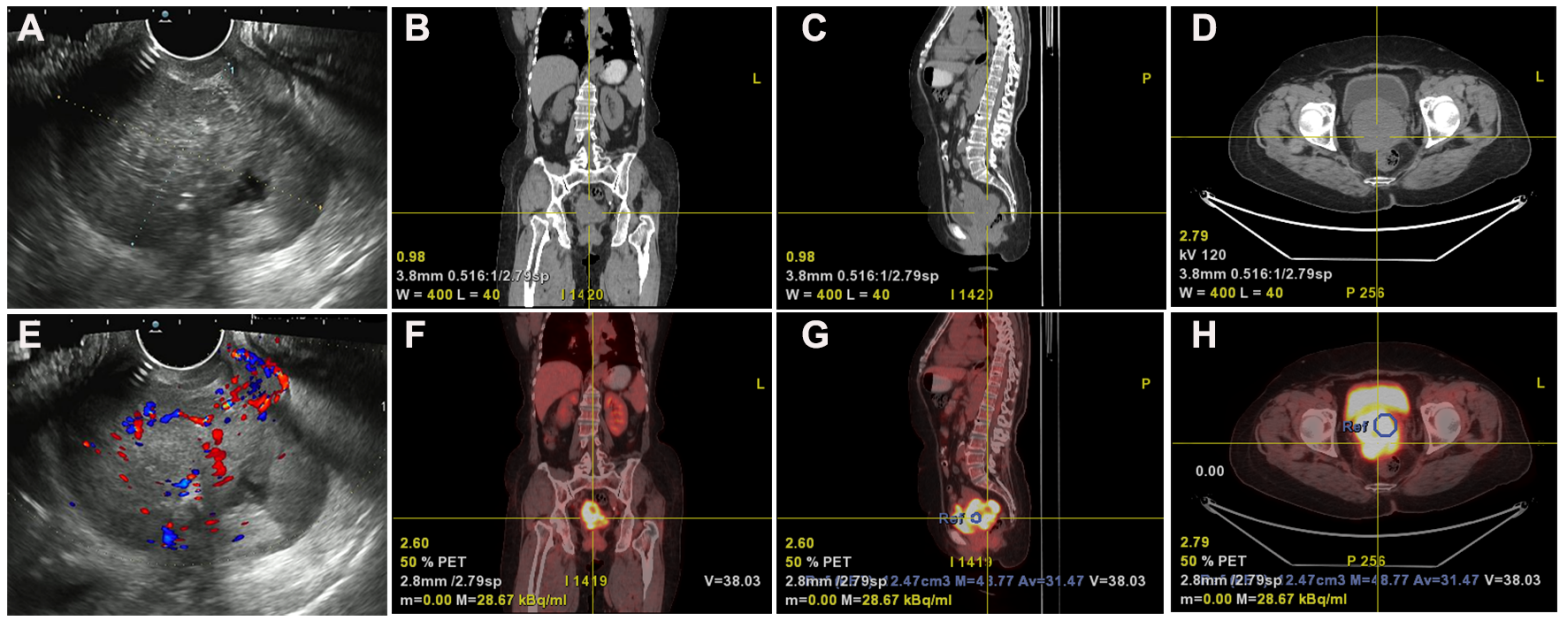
Ultrasound and PET-CT imaging revealed anatomical localization and metabolic activity. (A, E) Gynecologic ultrasound reveals an irregularly shaped, Multinodular cervical mass lesion measuring approximately 7.9 cm×6.8 cm×5.7 cm with partially indistinct boundaries, suggestive of confluence of multiple nodules. (B–D, F–H) PET-CT imaging demonstrates cervical soft tissue mass with necrosis, involvement of the uterine corpus and vagina, indistinct boundaries with bladder and rectum, and increased metabolic activity (SUVmax 16.8) in the Case of G-EAC.
